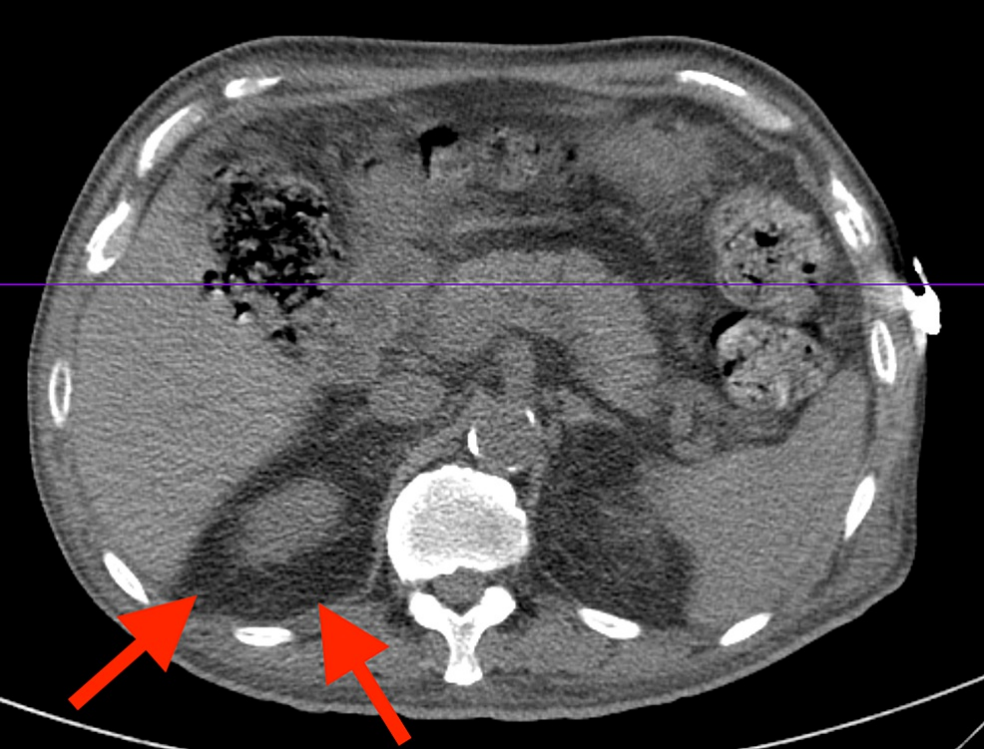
Abdominal and pelvic CT showing right-sided retroperitoneal hemorrhage (arrows) Subject to the imposed limitations, apparent resolution of the duodenal and pancreatic inflammatory changes. Thickened duodenum with mesenteric fat inflammatory changes suggesting duodenitis versus non-perforated peptic ulcer disease. Peripancreatic inflammatory changes are seen around the pancreatic head and are likely reactive; however, primary pancreatitis with reactive thickening of the duodenum cannot be excluded CT: computed tomography
